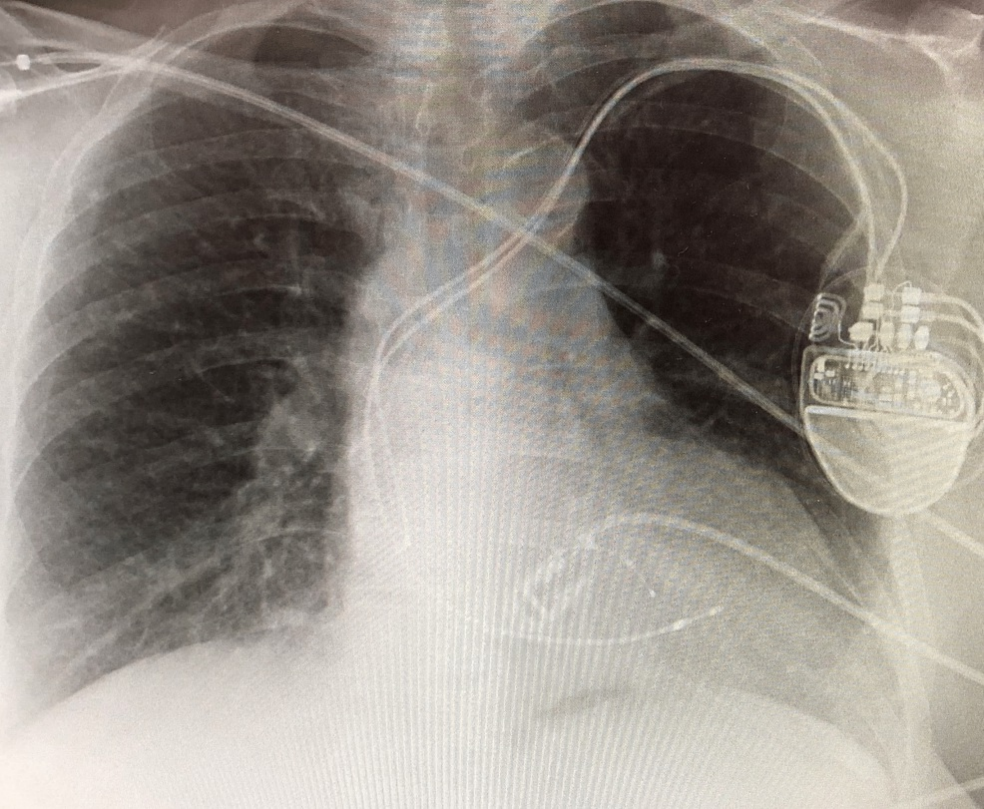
Chest radiograph showing CRT-P in situ CRT-P: cardiac resynchronization therapy pacemaker
